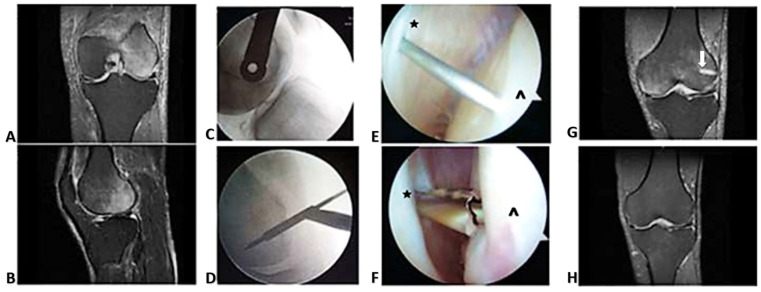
Migratory BME. (A) Coronal and (B) sagittal sections display high signal intensity in the lateral femoral condyle on the MRI T2 FS sequence. (C) Lateral and (D) AP X-ray views show fluoroscopic guidance of guide pin placement. (E,F) Arthroscopic guidance of pin and 4 mm cannulated drill tip through the joint capsule (*) to the femoral condyle (^). (G) BME observed in the medial femoral condyle on the coronal MRI T2 FS sequence. The previous decompression track is visible (arrow). (H) Coronal MRI T2 FS sequence showing resolution of high signal intensity in both condyles and healing of decompression tracks.
